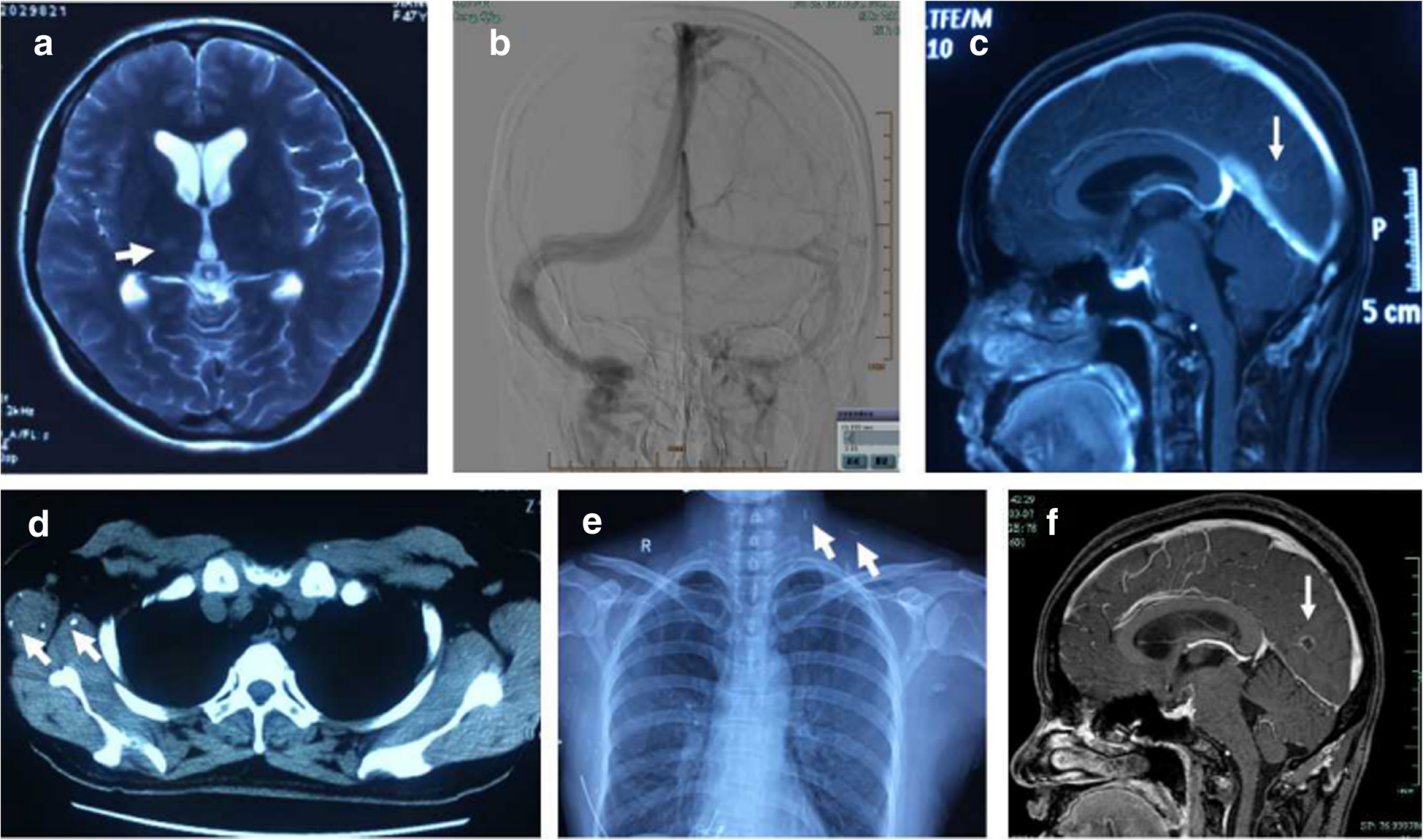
a The T2-weighted image showed a high signal of right thalamus. b DSA showed the probable underdevelopment on right lateral sinus while the venous system was smooth. c Cerebral MRI with contrast showed several small hyperintense lesions involving right cerebellar hemisphere and bilateral occipital, with ring enhancement of gadolinium. d The pulmonary CT showed multiple densities in the chest wall soft tissue. e Chest radiographs showed multiple calcification on the left side of the neck and right soft tissue of armpit chest wall. f The secondary MRI showed the nearly same small lesions with ring enhancement in the brain
